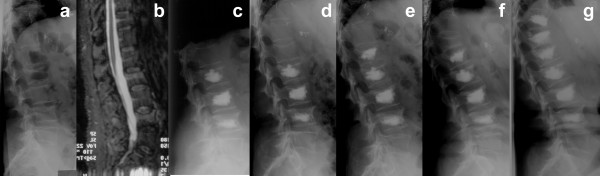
A 56-year old lady presented with painful compression fractures at L2, L3, and L4 due to corticoid-induced secondary osteoporosis (a, b). As pain did not improve during non-surgical therapy for 6 weeks, kyphoplasty at L2–L4 was performed (c). Two weeks postoperatively the patient reported again severe back pain. The radiographs revealed an adjacent compression fracture at L1 (d). Therefore a kyphoplasty at L1 was performed (e). One month later the patient presented again with severe thoracolumbar back pain, because of an adjacent fracture at T12 (f). After kyphoplasty of T12 and prophylactic kyphoplasty of T11 the patient remained without further fractures (g).
